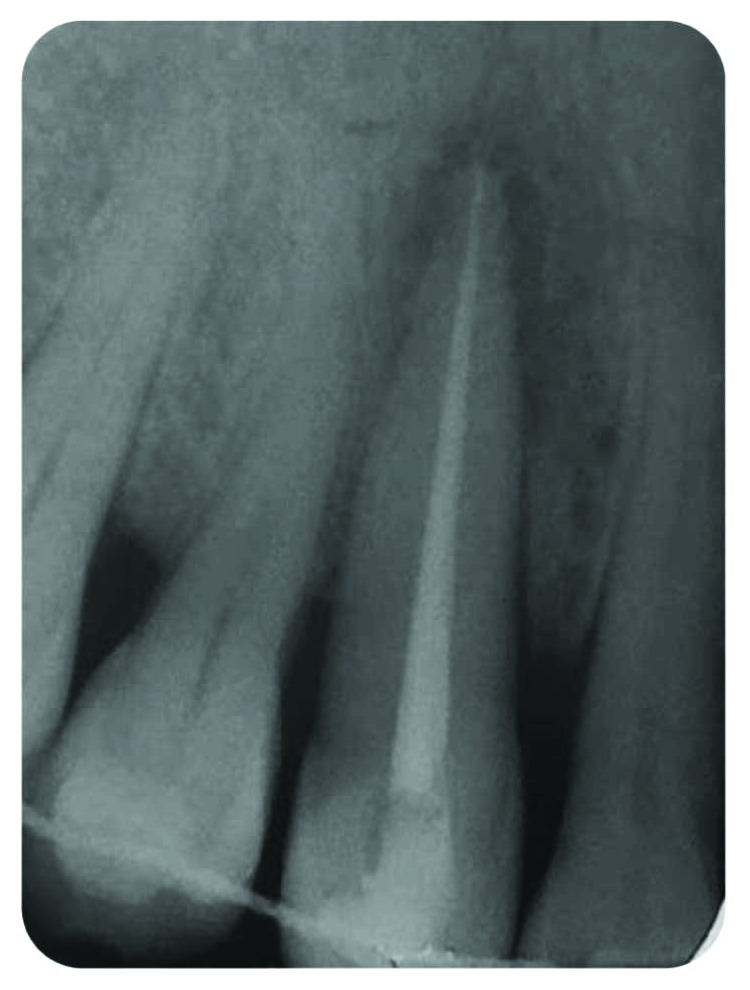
18 months' recall radiograph.
